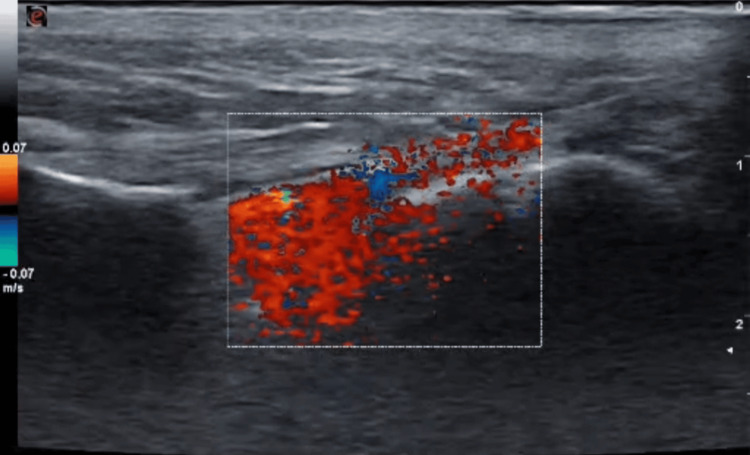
Color Doppler showing an appropriate flow of the local anesthetic
